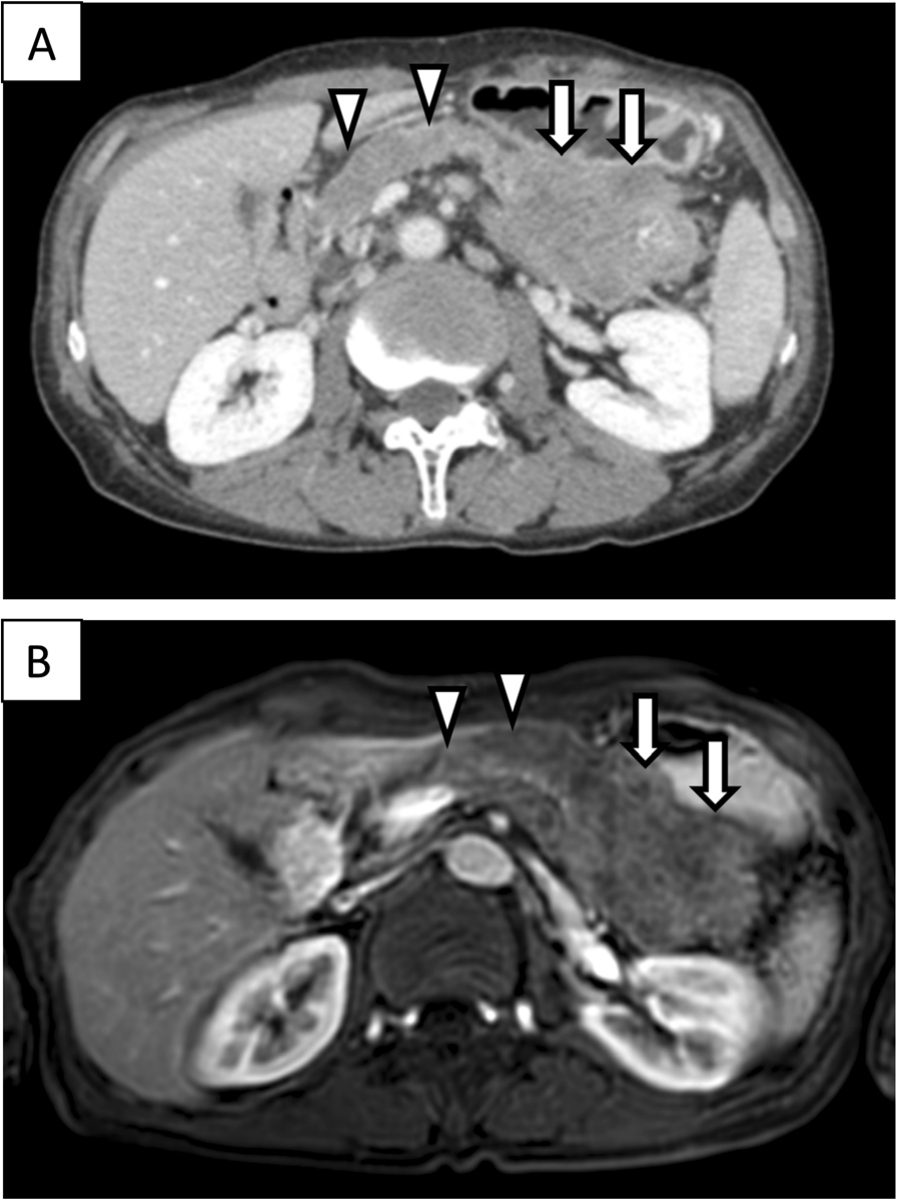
Episode 1: primary pancreatic tumor. Diffuse pancreatic mass in a 67-year-old man. Contrast-enhanced computed tomography (CT, a) scan and magnetic resonance imaging (MRI, b) showed a hypovascular pancreatic mass located mainly in the pancreatic tail (arrows) and involving the entire pancreas (arrow head)
